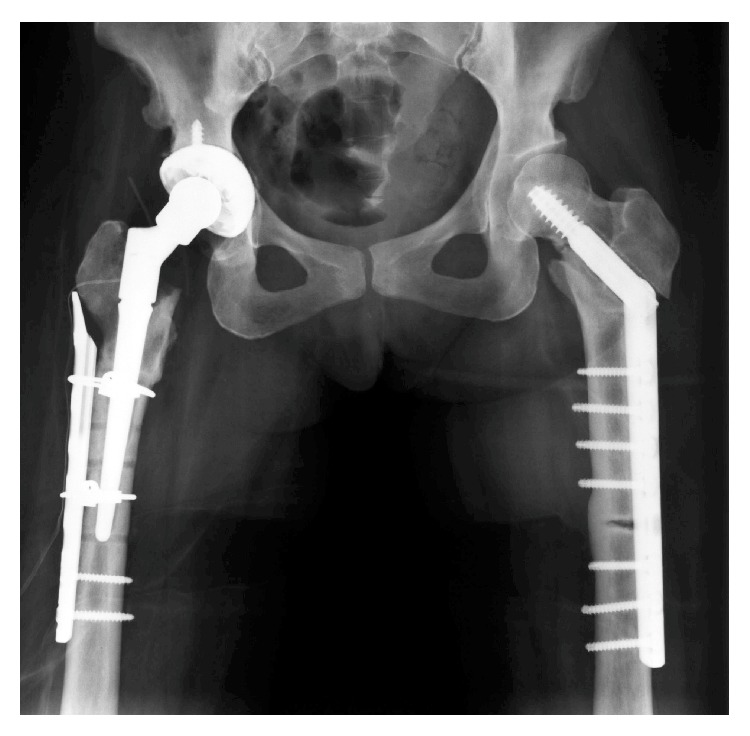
Radiograph after total hip arthroplasty with cable and plate fixation.
